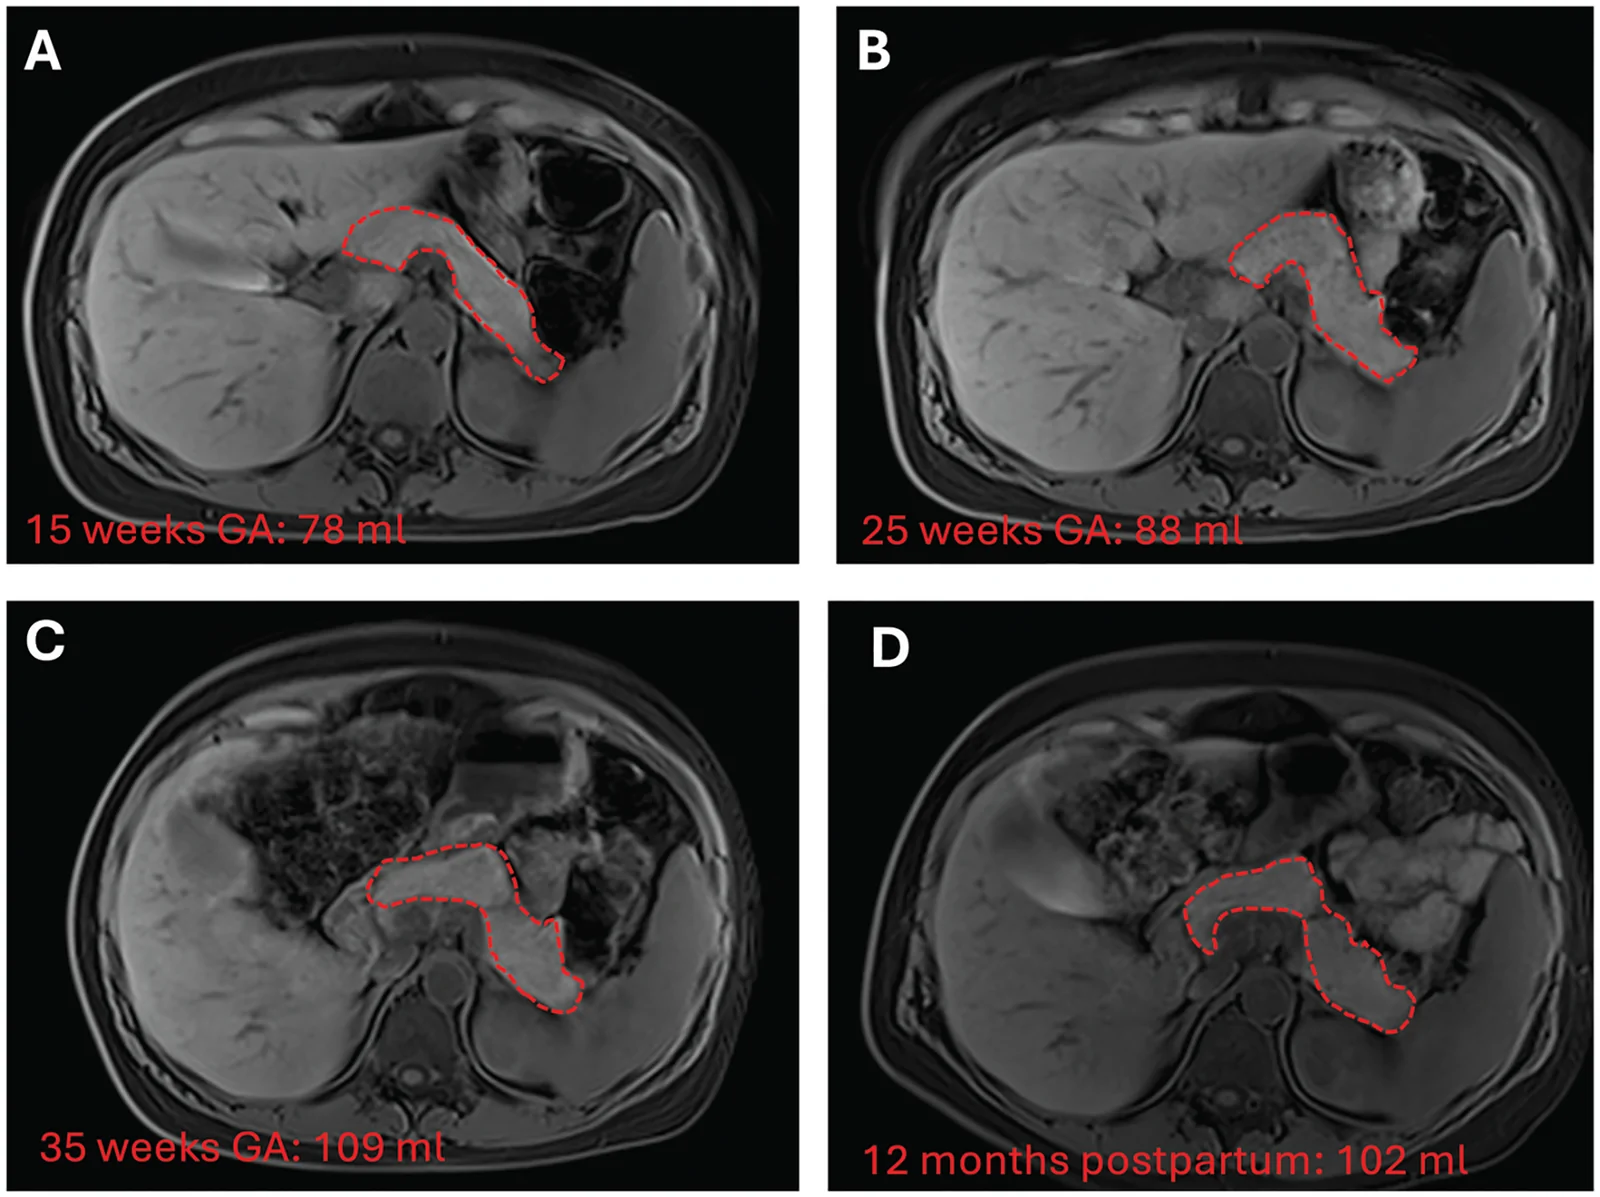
Longitudinal MRI scans of a representative study participant acquired at (A) 15 weeks’ gestation, (B) 25 weeks’ gestation, (C) 35 weeks’ gestation, and (D) 12 months’ postpartum. The pancreas is outlined in red on each axial MRI slice and the pancreas volume is noted on each image. The pancreas volume increased over the course of pregnancy from 78 mL to 109 mL and declined postpartum to 102 mL.
